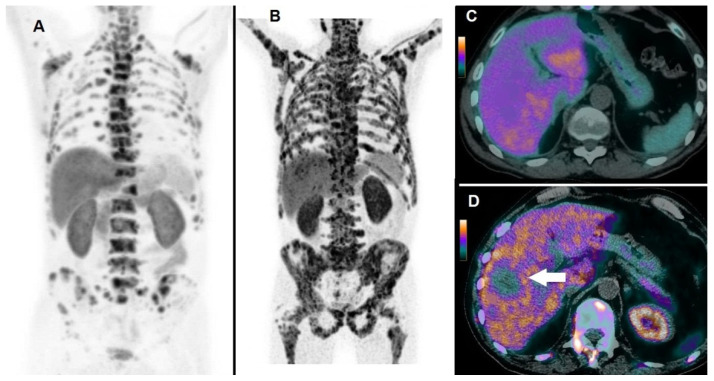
A 66-year-old patient affected by mCRPC, previously treated with abiraterone acetate and docetaxel, then submitted to ^223^Ra-therapy. ^18^F-choline PET/CT acquired at baseline demonstrated multiple tracer uptake sites in bones, as evident in Whole Body (A); baseline pre-treatment parameters were as follows: PSA = 157 ng/mL; the number of lesions > 20; TLA = 3969.9 g. After 3 cycles, ^223^Ra-therapy was discontinued due to deterioration of patient’s performance status and increased PSA (i.e., 289 ng/mL). ^18^F-choline PET/CT acquired after 3 cycles of ^223^Ra-therapy demonstrated an impressive progression of skeletal disease, as shown by Whole Body (B). ^18^F-choline PET/CT fused images of the liver acquired at baseline did not show abnormal tracer uptake (C), while post-treatment axial slices demonstrated the appearance of a partially necrotic metastasis in the VIII/VII hepatic segment (D, white arrow). The patient’s OS resulted in 7 months.
